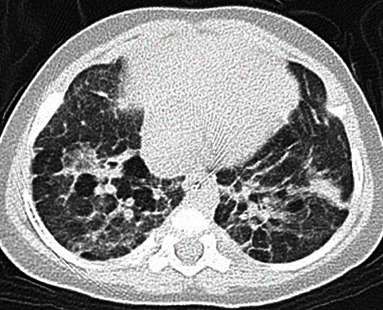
Axial CT on the 244th DOL of case 2.
